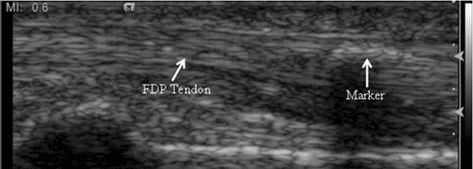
Ultrasound image of finger area in cadaver
